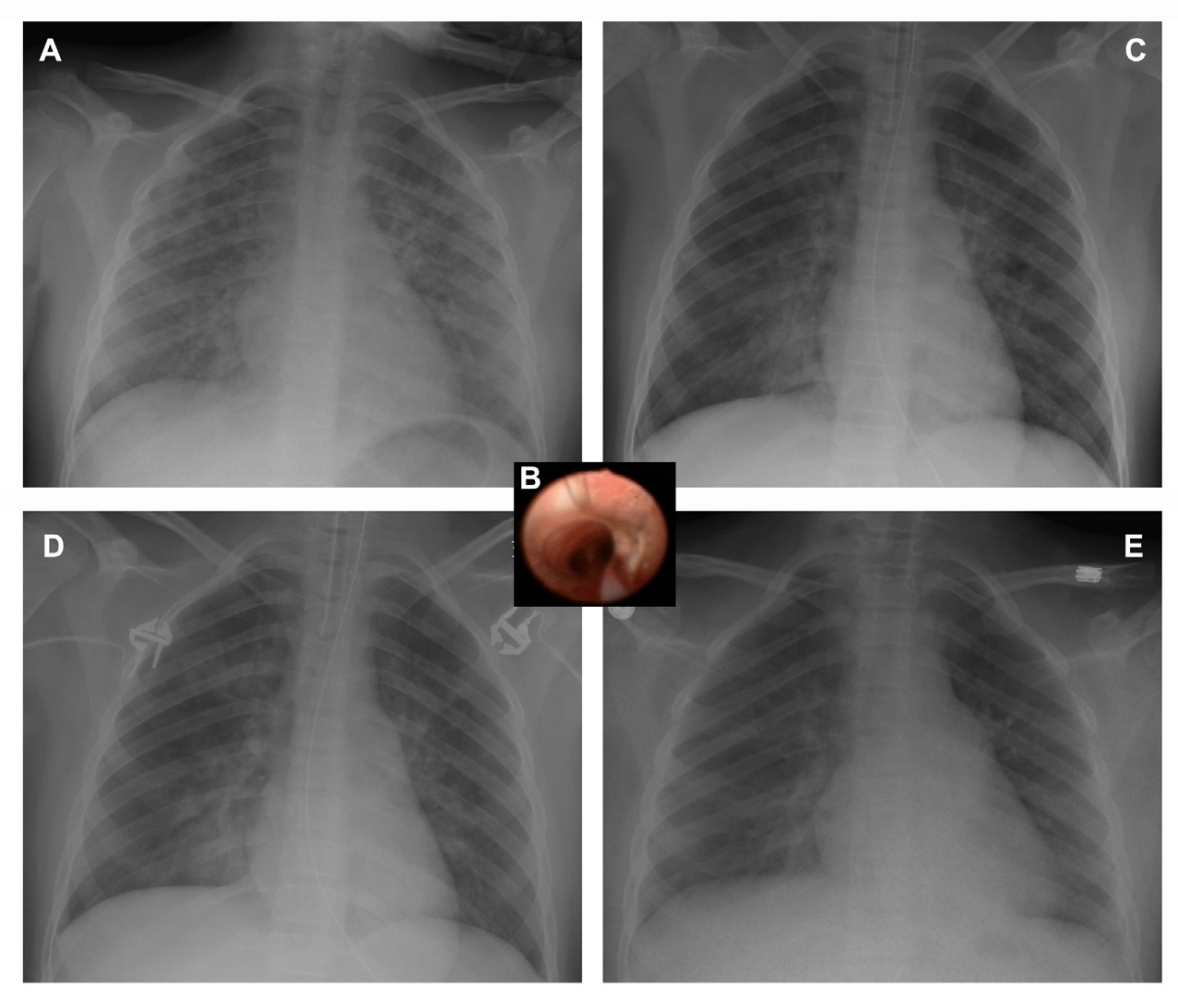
Chest radiographic images of a fourteen year-old patient. Onset of pulmonary edema at hour 0 (panel
